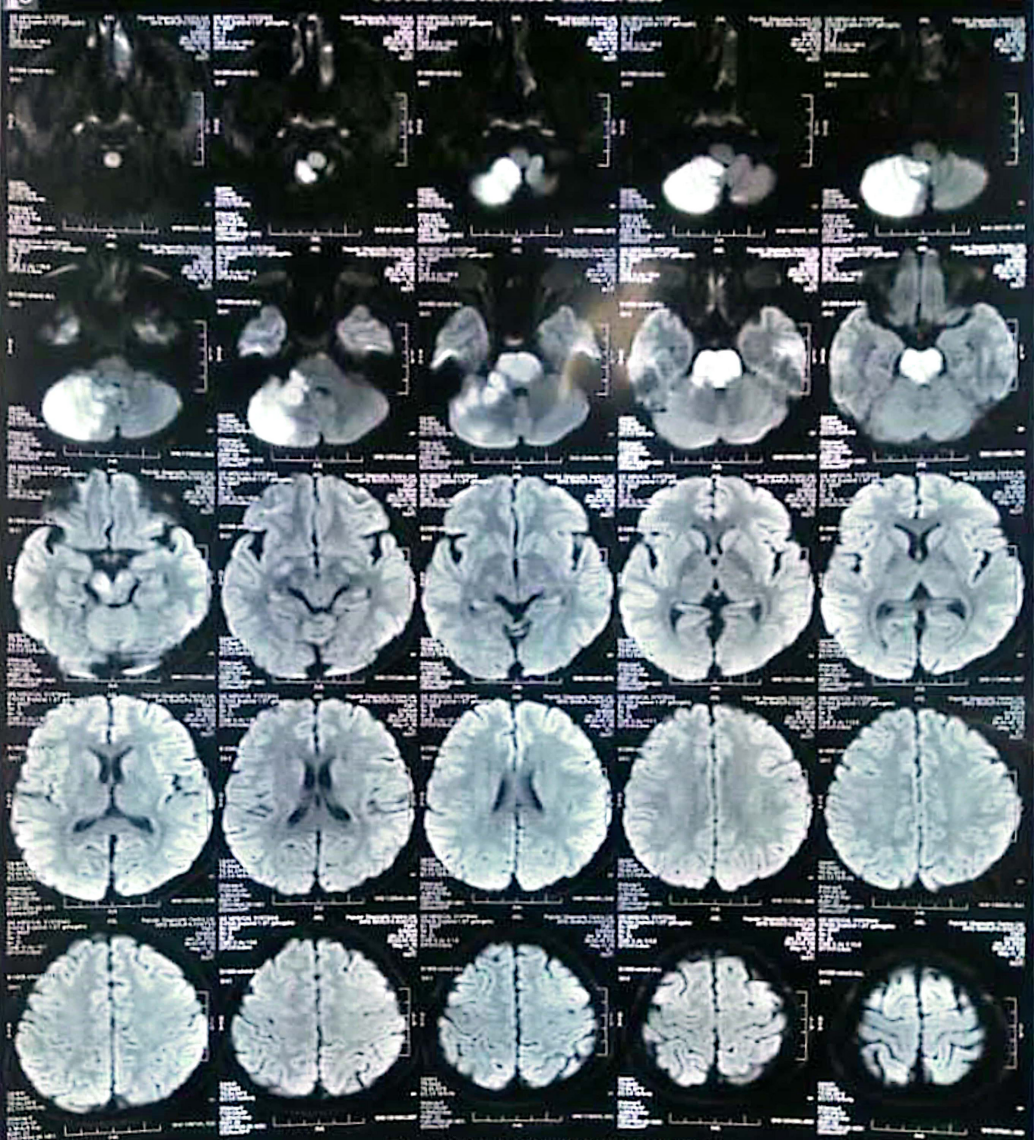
MRI brain showing acute infarct in right cerebellar hemisphere and pons.
